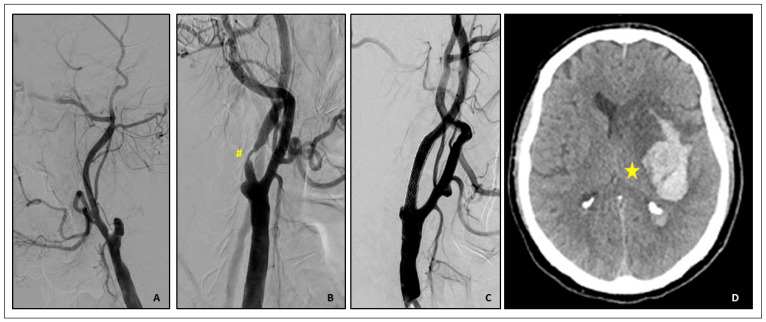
(A–C) Digital subtraction angiography (DSA). (A). Right internal carotid artery occlusion. (B). Tight left ICA stenosis (yellow blanket). (C). Final result after Carotid WallStent 9.0/30 mm (Boston Scientific, USA) deployment and post dilatation. (D) The brain CT scan, performed 2 h following carotid revascularization, shows a large right basal ganglia haemorrhage (star).
